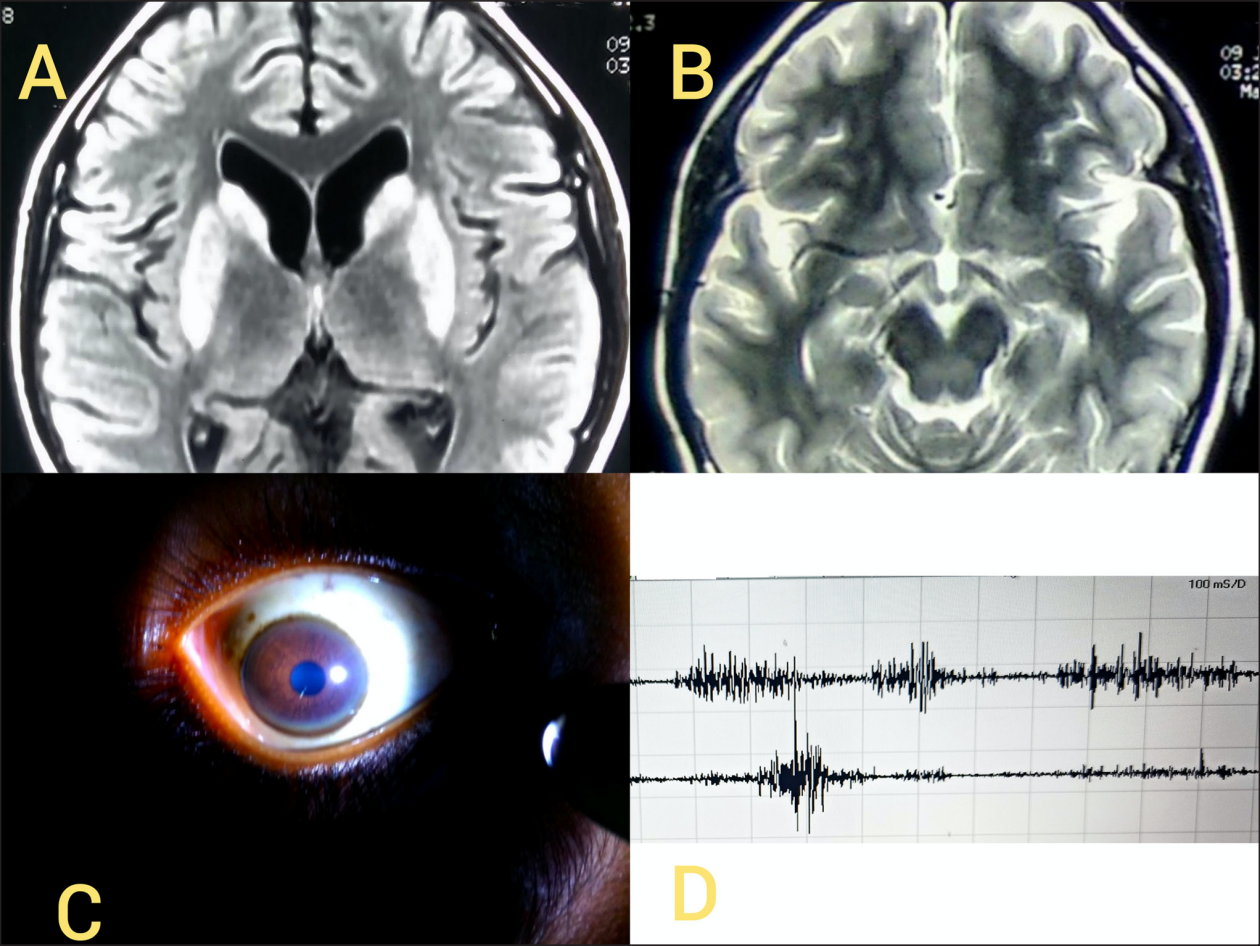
(A) MRI Axial T2 weighted fluid attenuation inversion recovery (FLAIR) sequence showing symmetric hyperintensity of bilateral caudate and putamen as well as (B) hyperintensities of midbrain. (C) Demonstration of brown coloured KF ring in the patient (D) Surface EMG of right upper limb of patient with electrodes over Extensor digitorum communis (EDC) and flexor digitorum superficialis (FDS) showing alternating bursts of motor unit action potentials (MUAPs).
